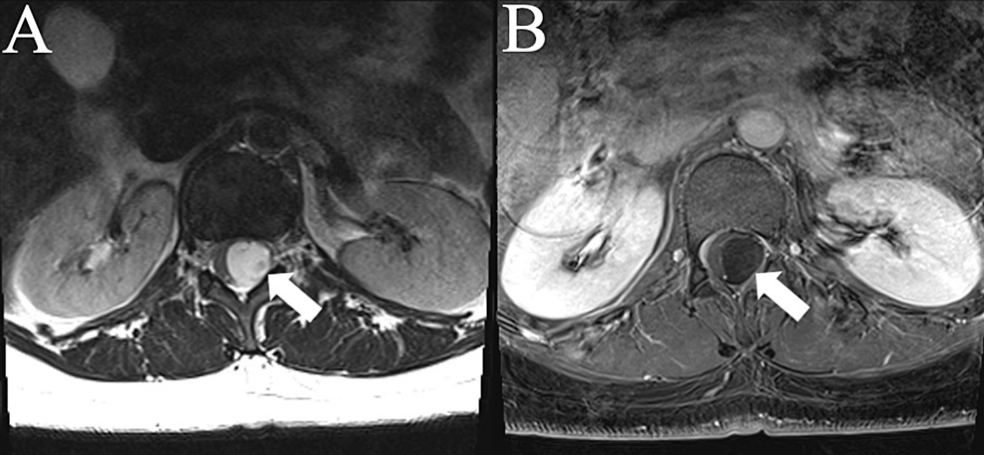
Axial MRIs demonstrating the T12-L1 intradural extramedullary lesion The axial MRIs demonstrate the T12-L1 lesion (white arrow) found on imaging. (A) is an axial T2 MRI without gadolinium. (B) is an axial T2 MRI with gadolinium, showing no evidence of enhancement.
